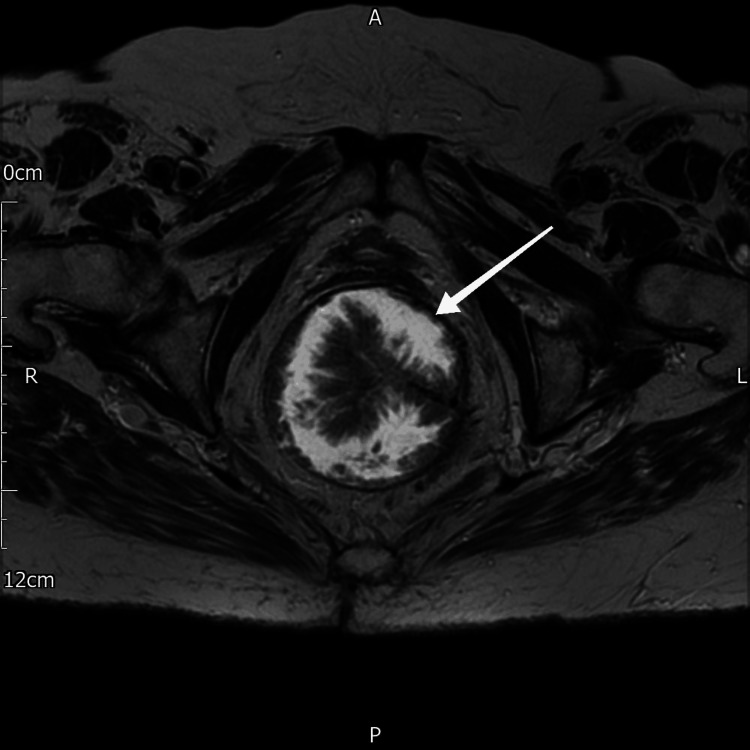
MRI image of the rectal polyp The white arrow indicates the tumor, with finger-like villi projecting into the rectal lumen.
